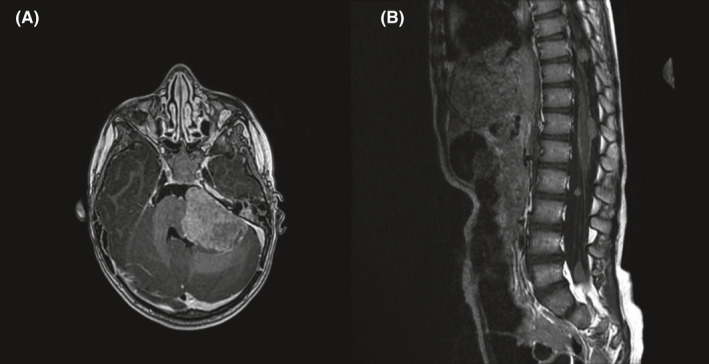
Trilateral retinoblastoma diagnosed at age 37 months. MRI shows (A) a large tumor (3.5 × 5.5 × 5.5 cm) in the cerebellopontine angle and subependymal tumor elements in the rim of the lateral ventricle anterior horns and (B) several drop metastases in the thoracolumbar spinal canal
